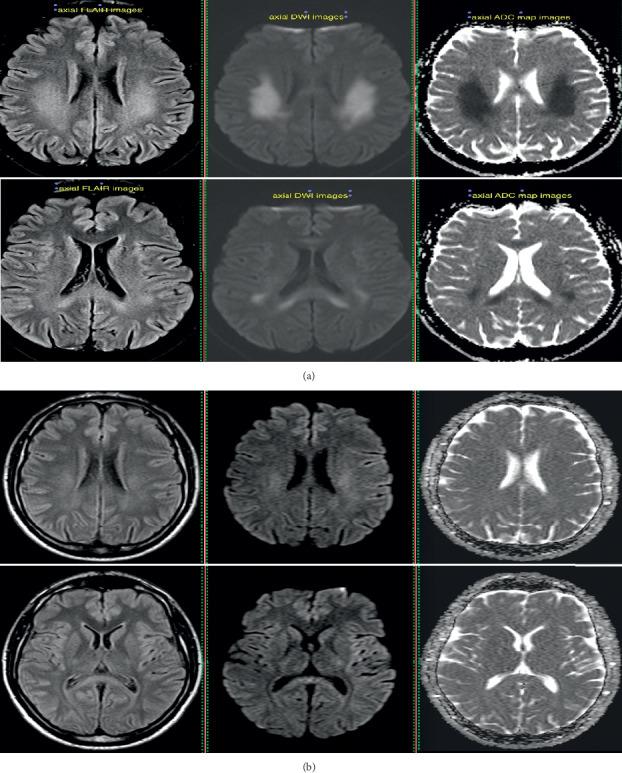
(a) Axial fluid-attenuated inversion recovery (FLAIR) and diffusion-weighted magnetic resonance imaging (DWI) upon symptom presentation showed diffuse symmetric deep white matter involvement of the centrum semiovale posterior frontal and parietal, with T2/FLAIR hyperintensity and restricted diffusion (first row of images), which extend continuously to the splenium of the corpus callosum (second row of images). (b) Follow-up MRI 1 month after presentation showed significant symmetric improvement of the findings on FLAIR and DWI images. The FLAIR abnormalities had improved both in volume and intensity; subtle increased signal could still be traced on DWI images, with no actual restricted diffusion on ADC map images.
